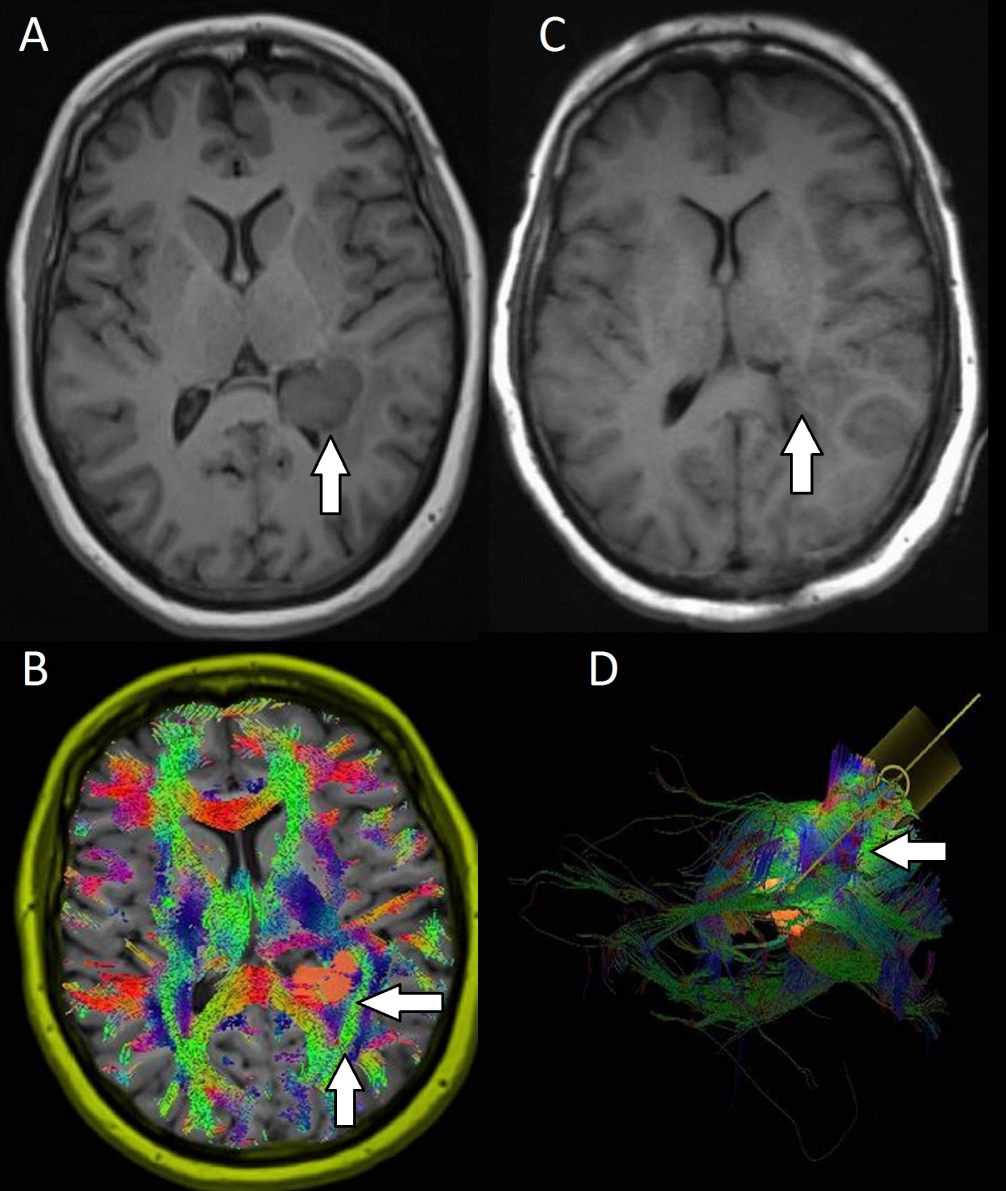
Preoperative and postoperative images for patient four with a left atrial meningioma A: Preoperative MRI showed a diffusion-restricting mass (arrow) in the atrium of the left ventricle, causing mass effect in the left temporoparietal region. B: DTI showed that the lesion was displacing the left ILF (partially visualized, leftward arrow), front-occipital (not visualized here) and optic radiation (partially visualized, upward arrow) fibers. C: Postoperative images showing gross total resection of the mass, which was determined to be a WHO Grade I meningioma. D: A variant of the superior parietal lobule approach was designed to engage the association fibers in a parafascicular fashion (arrow) so as to minimize injury.
